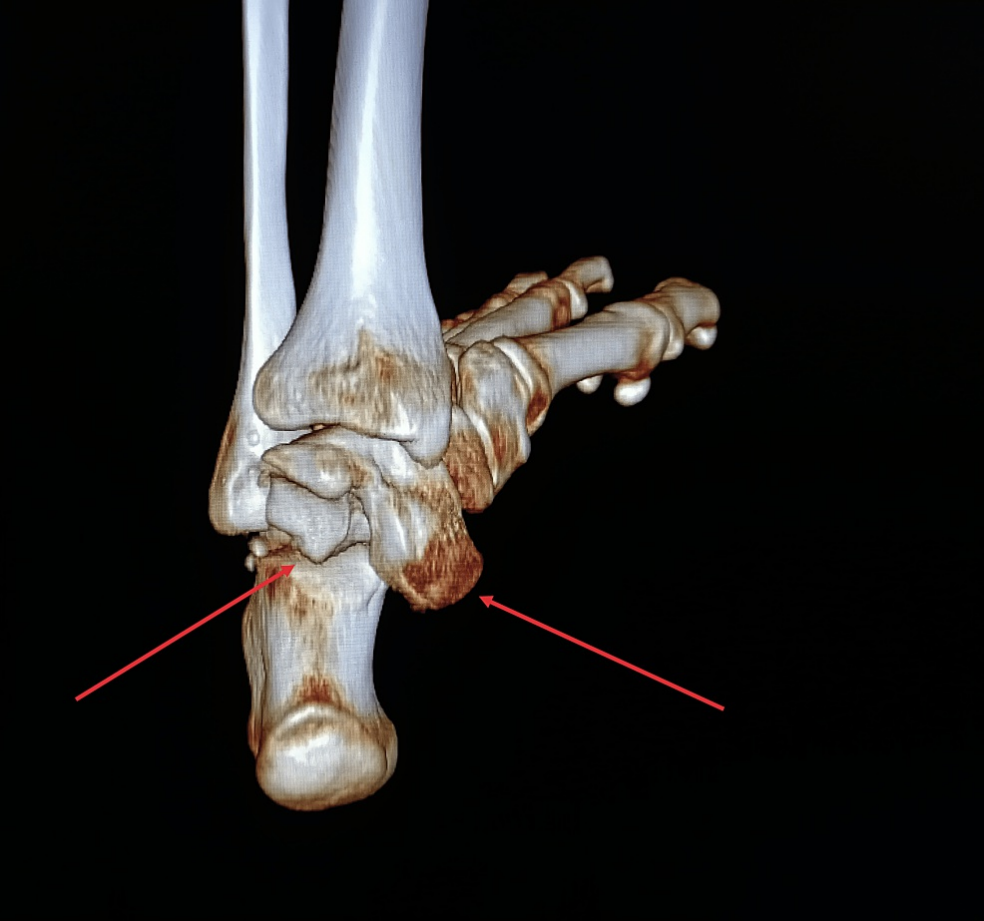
3D CT-scan reconstruction of the left ankle. The arrows designate the subtalar dislocation and the medial rotation of the talus.
